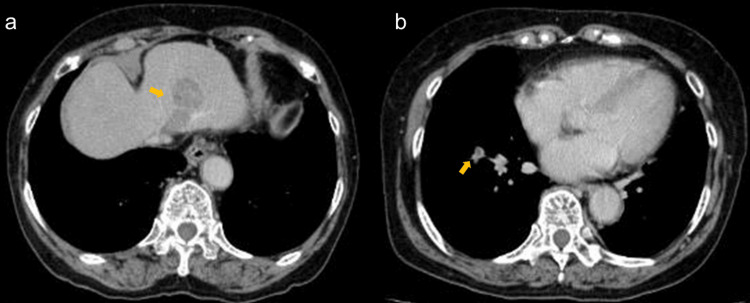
Radiologic findings before surgery. (a) Enhanced computed tomography showed heterogenous 28 mm and 17 mm low-density areas as suspected hepatic tumors and hepatic vein tumor embolus. (b) Enhanced computed tomography showed pulmonary thromboembolism in a right lung field (arrow).
